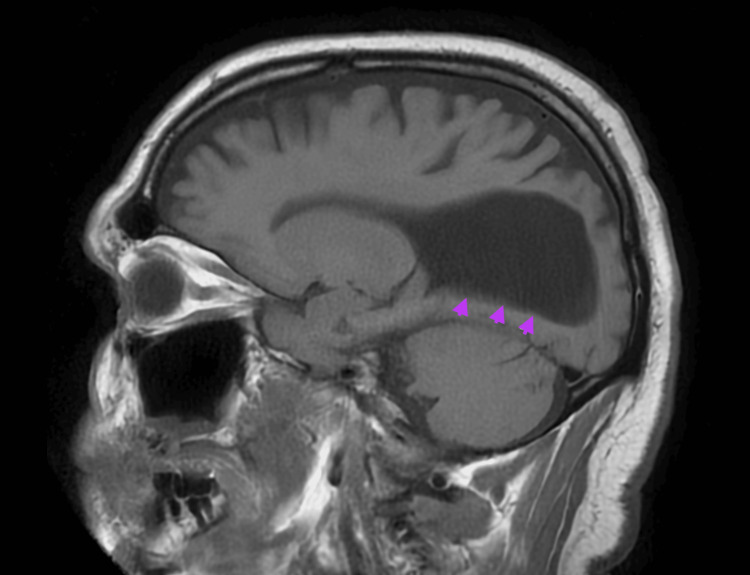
T1 MRI brain sagittal view of the “sunrise” sign characteristic of corpus callosum agenesis.
